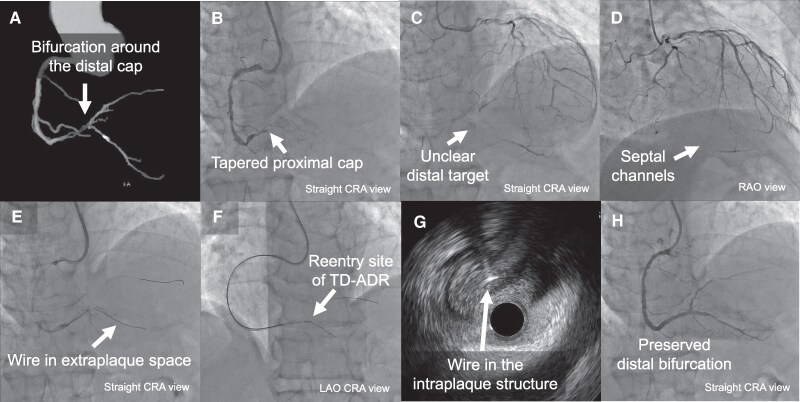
Images of patient 2: (*A*) CT angiogram showing a bifurcation around the distal cap. (*B*) Right coronary angiography demonstrating a tapered proximal cap. (*C*) Left coronary angiography showing an unclear distal target. (*D*) Left coronary angiography showing septal collateral channels. (*E*) A guidewire went extraplaque plane of the posterior descending artery. (*F*) Re-entry site of tip detection-antegrade dissection and re-entry (TD-ADR). (*G*) A Conquest Pro 12ST guidewire in the intraplaque structure. (*H*) Final angiogram showing a preserved distal bifurcation.
